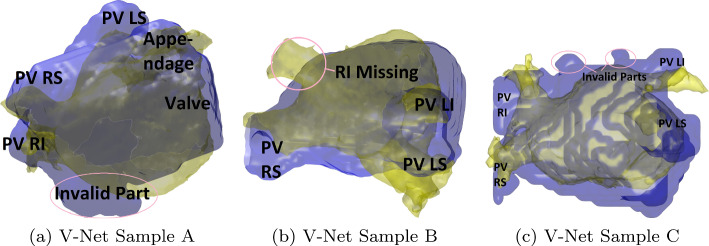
Invalid anatomy in V-Net reconstructions. The CT is in yellow, reconstruction is in blue, invalid anatomy is circled in pink
